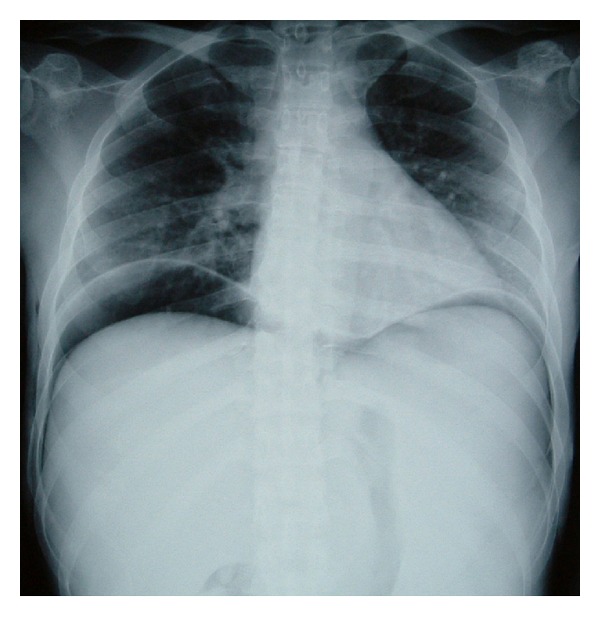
Chest radiograph at the initial diagnosis showed bilateral hypodiaphragmatic free air.
